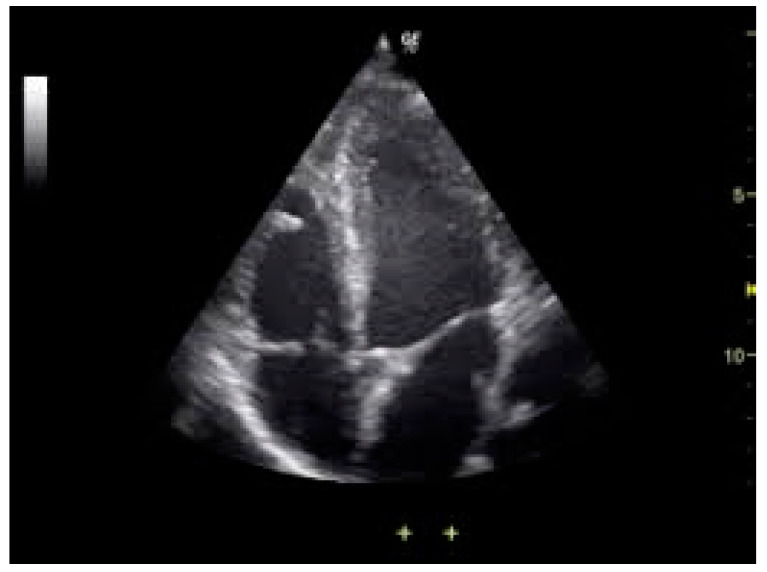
Postoperative echocardiography showed normal findings.
